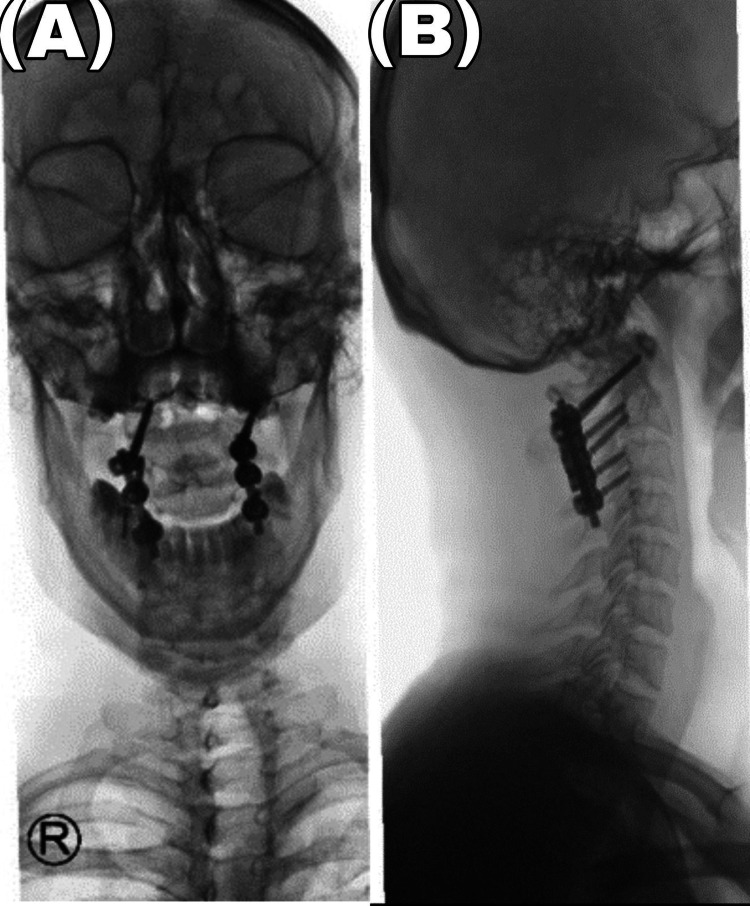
Lateral atlantoaxial spine radiograph during follow-up (a) Open mouth, (b) Showing the satisfactory alignment of C1 and C2 and good positioning of the screw
